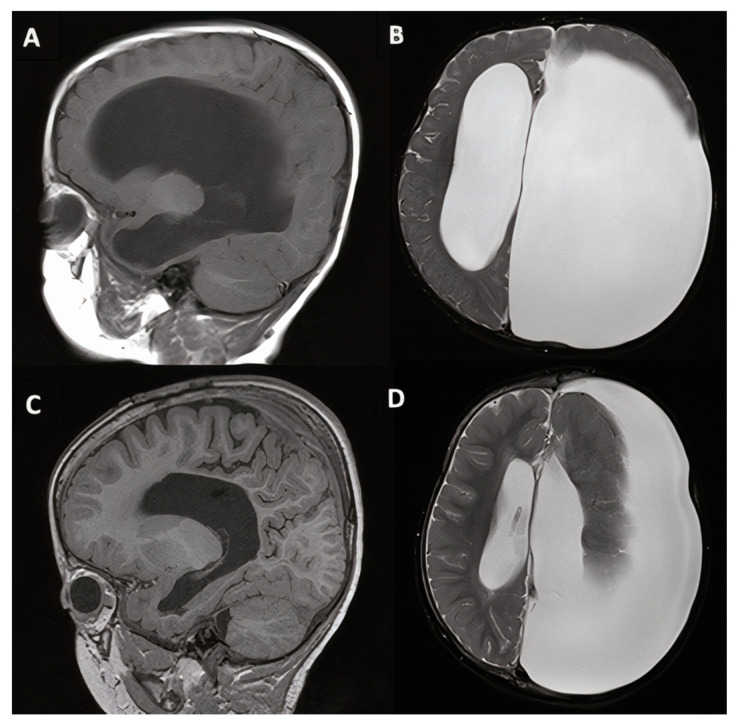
18-month-old male patient with left-sided porencephalic cyst secondary to intrauterine cerebral ischemia (case #9). Pre-operative (A,B) and 2 years post-operative (C,D) T1-weighted right-sided sagittal and T2-weighted axial magnetic resonance imaging (MRI), demonstrating ventriculomegaly as well as left-sided porencephalic cyst.
